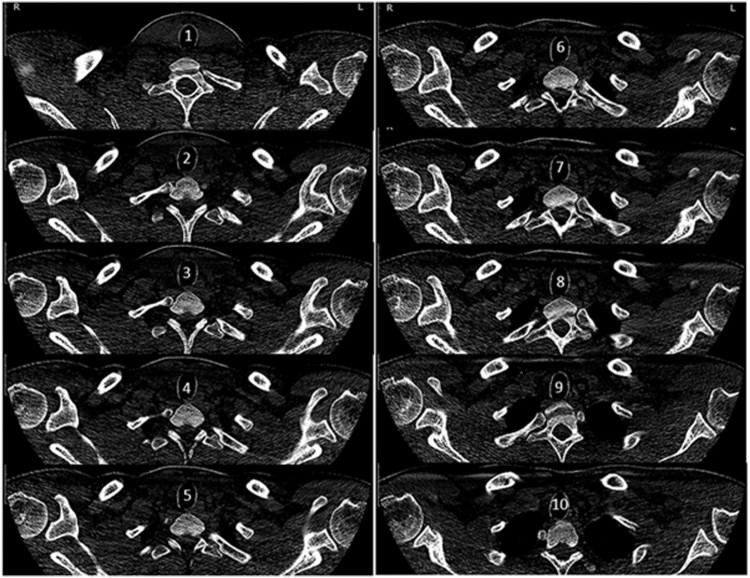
Computed tomography of the chest conducted on the day of trauma as part of standard polytrauma assessment. The image revealed no abnormal densities within or traversing the bicipital grooves bilaterally.
